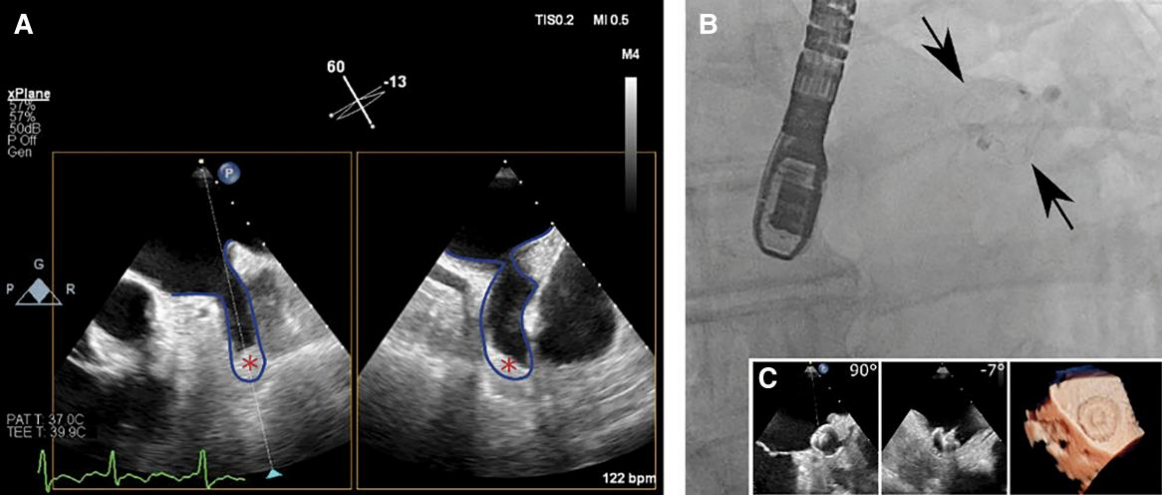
(*A*) X-plane transoesophageal view of the left atrial appendage showing a left atrial appendage thrombus. Blue line: left atrial appendage walls. Asterisk: thrombus. (*B*) Left atrial appendage closure procedure: final radiographic image of the left atrial appendage occluder placement (Watchman device). (*C*) X-plane transoesophageal view (right) and 3D view (left) showing the deployed and released Watchman device in left atrial appendage.
